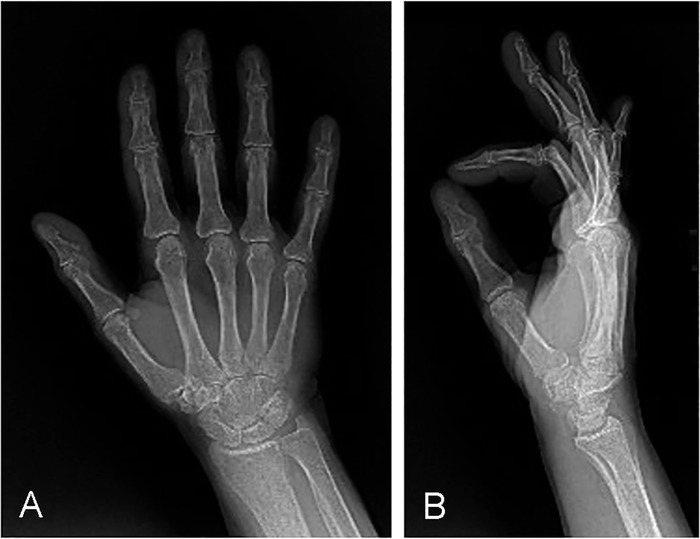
(A) Posteroanterior and (B) lateral radiographs show mild degenerative joint disease of the interphalangeal and carpometacarpal joints of the thumb.
